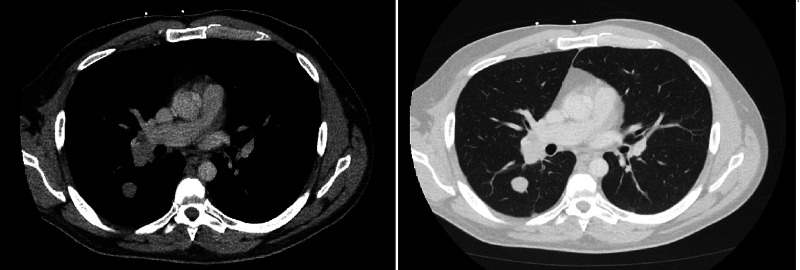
CT scan of the lung with contrast showing right hilar lymph node and right upper lobe mass
